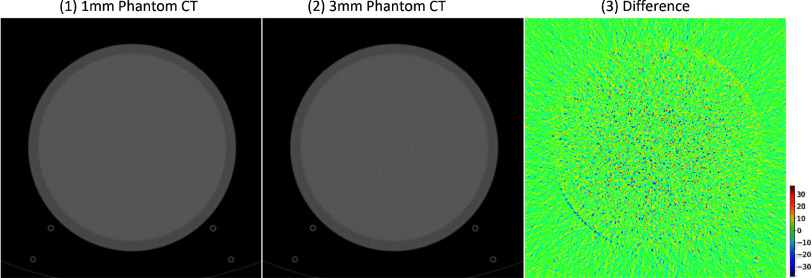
The full dose CT images reconstructed from B30 kernels acquired with different slice thickness and their differences.
